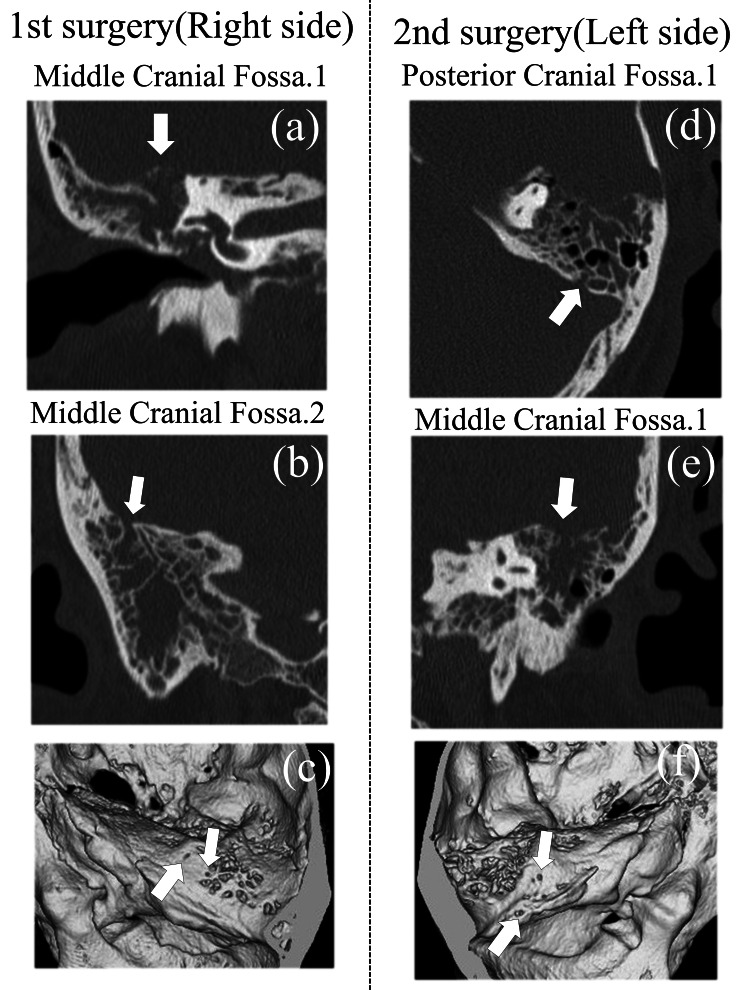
Preoperative computed tomography (CT) findings (a) A 2-mm bone defect in the middle cranial fossa at the level of the superior semicircular canal, (b) a 2-mm bone defect in the middle cranial fossa, slightly lateral to the first defect, (c) three-dimensional CT showing small bony defects in the right middle cranial fossa, (d) a 2-mm bone defect in the posterior cranial fossa, (e) a 2-mm bone defect in the middle cranial fossa, (f) three-dimensional CT showing small bony defects in the left posterior and middle cranial fossae.
